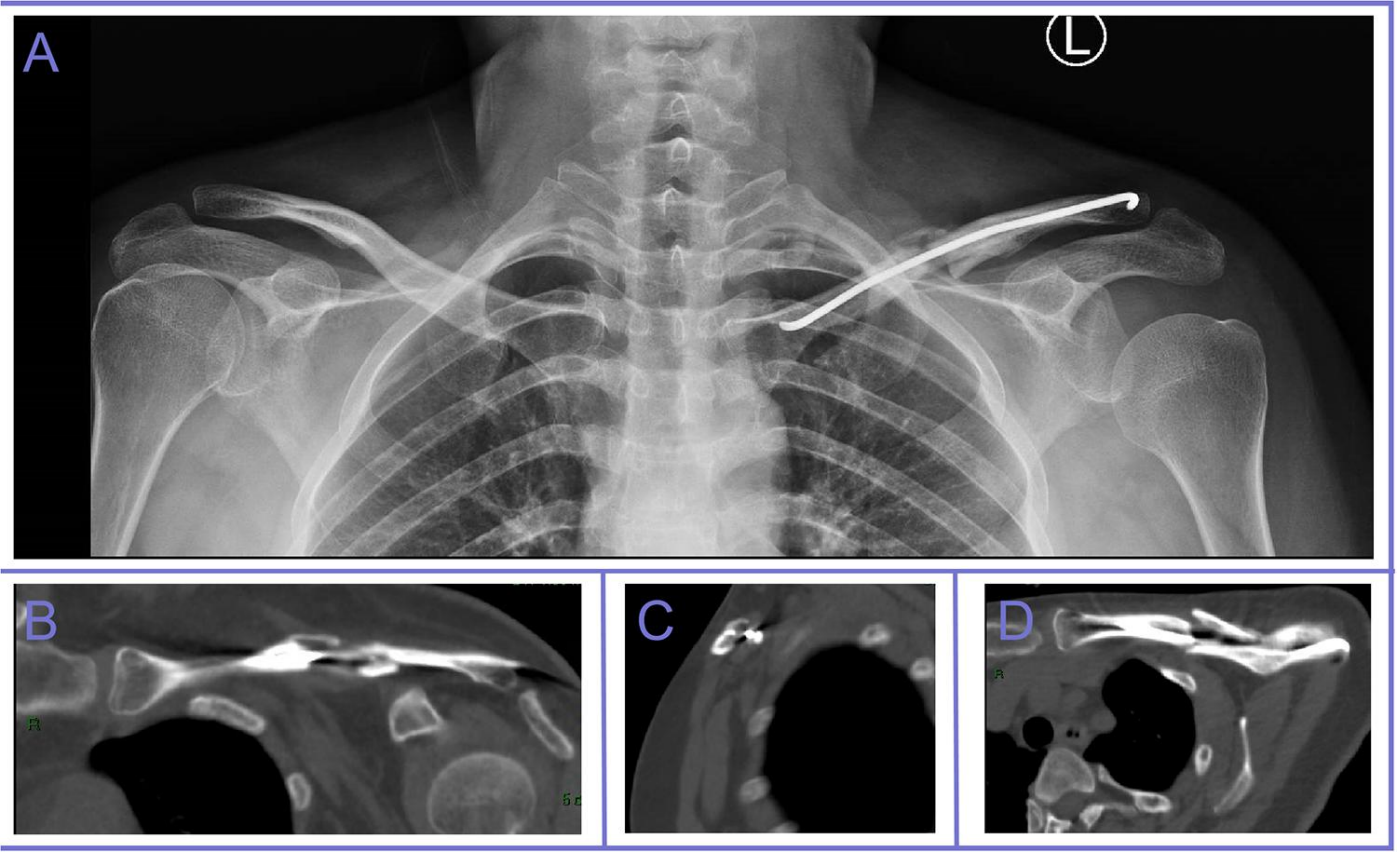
x-ray and CT imaging revealed significant bone callus formation at the fracture site.
